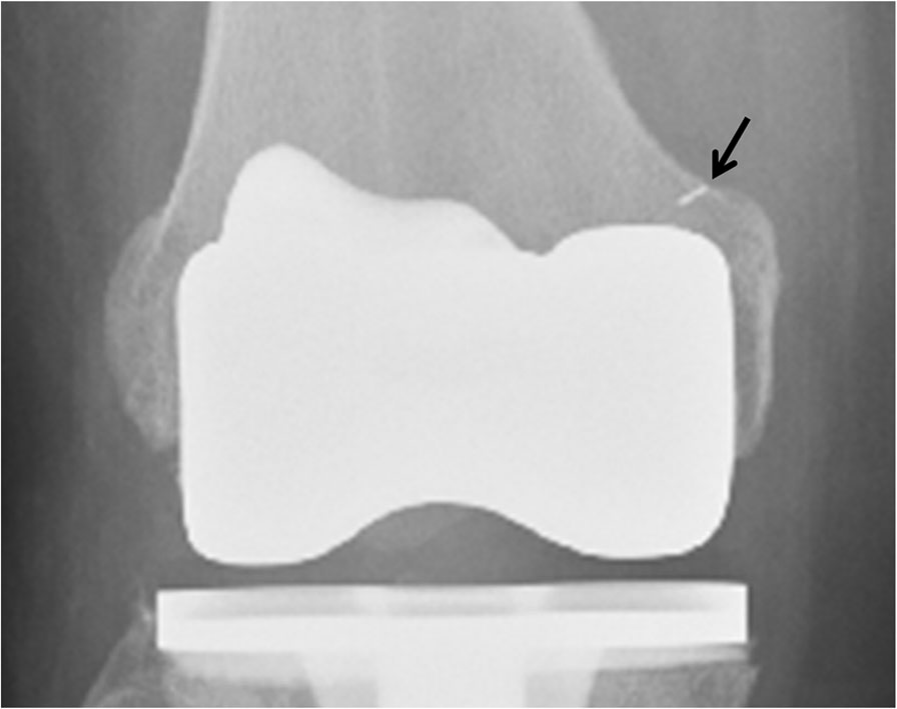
Metal marker on a radiograph and its relationship to the inflection point (arrow). The metal marker was implanted on the adductor tubercle during surgery. On the postoperative radiograph, the marker is located almost at the inflection point
